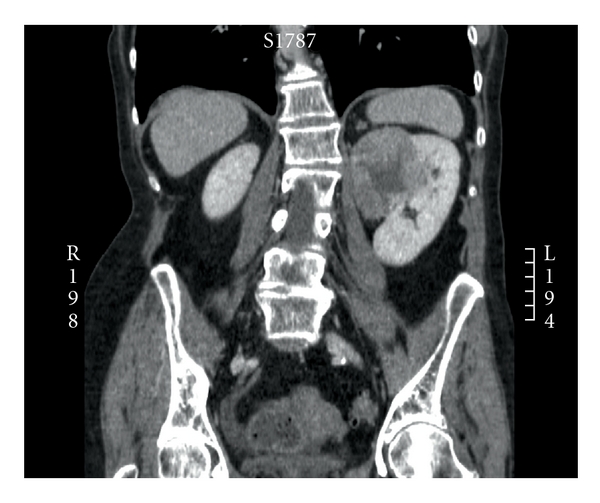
CT coronal image of the kidney tumor. The upper pole tumor lies medially in the left kidney.
